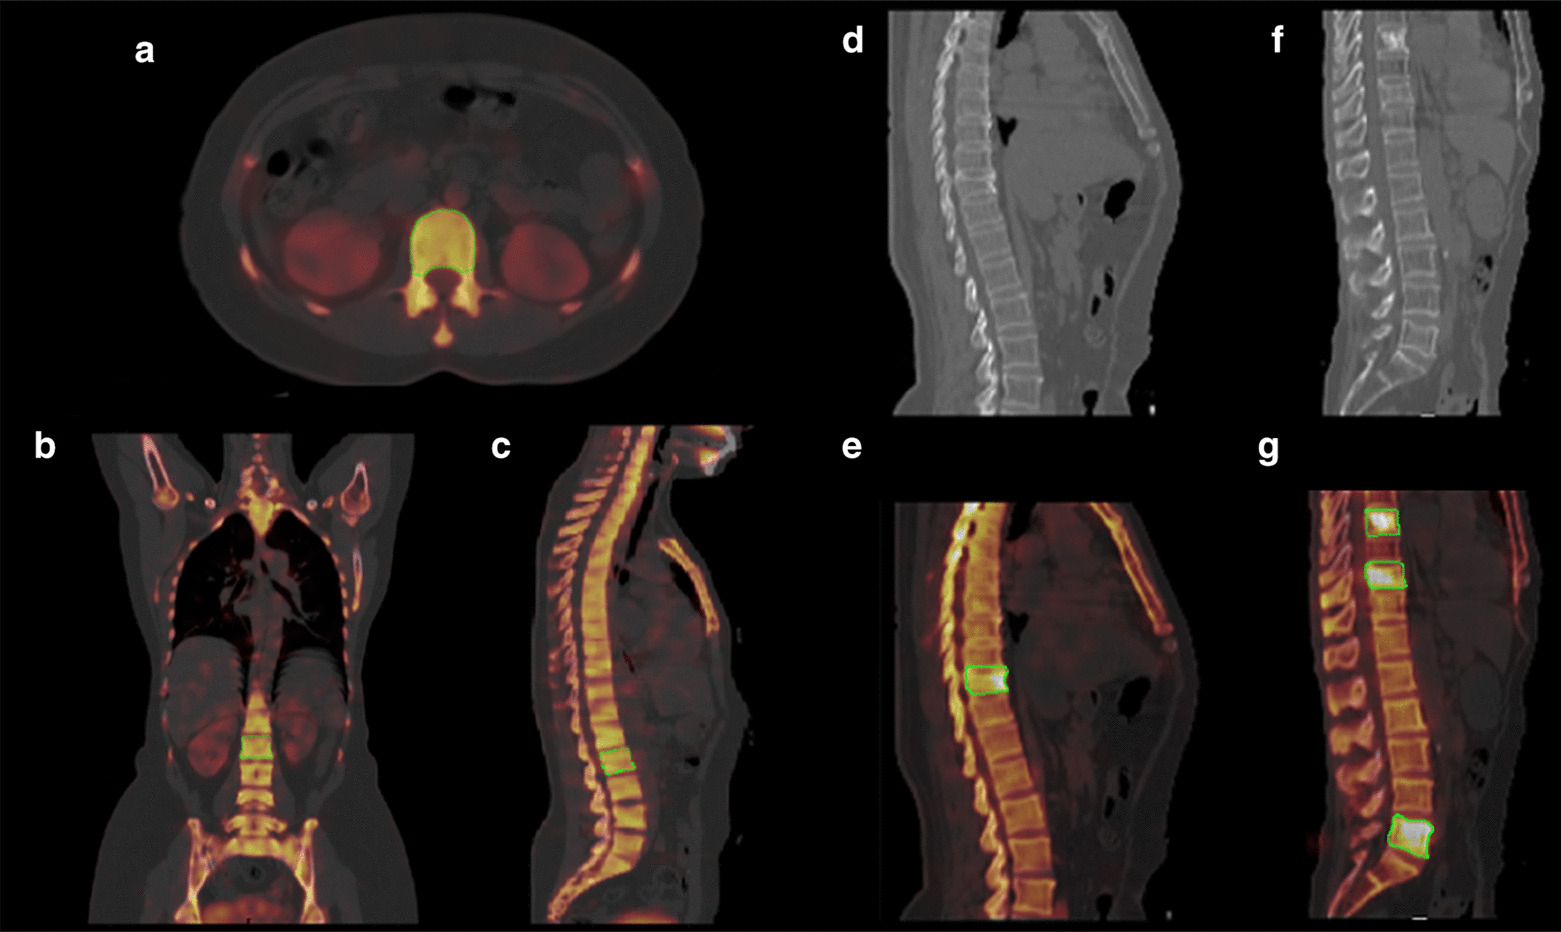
Representative SPECT/CT images of normal vertebrae, benign and malignant bone lesions. Transaxial (a), coronal (b) and sagittal (c) SPECT/CT images of a patient with normal thoracic and lumbar regions. The ellipses depicted the VOI of L2(using Siemens 3D Isocontour), SUV_max_ = 6.75; Sagittal CT (d) and SPECT/CT (e) images of a patient with T10 compression fracture (SUV_max_ = 13.06); Sagittal CT (f) and SPECT/CT (g) images showed a patient who had surgery of prostate cancer 5 years ago with T8, T10, and L5 bone metastases (SUV_max_ are 19.76, 18.27, 19.69 respectively)
